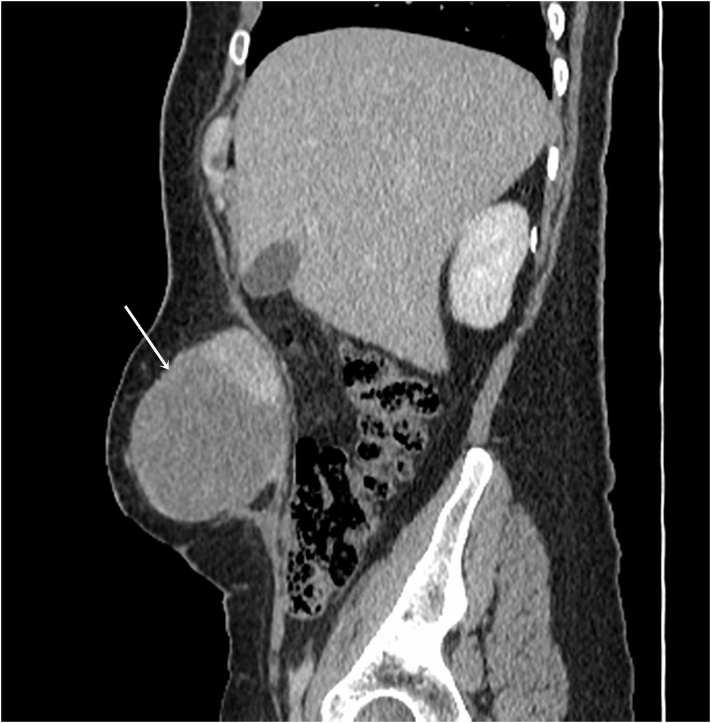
CT scan in the sagittal plane showing the mass of the abdominal wall (arrow).
